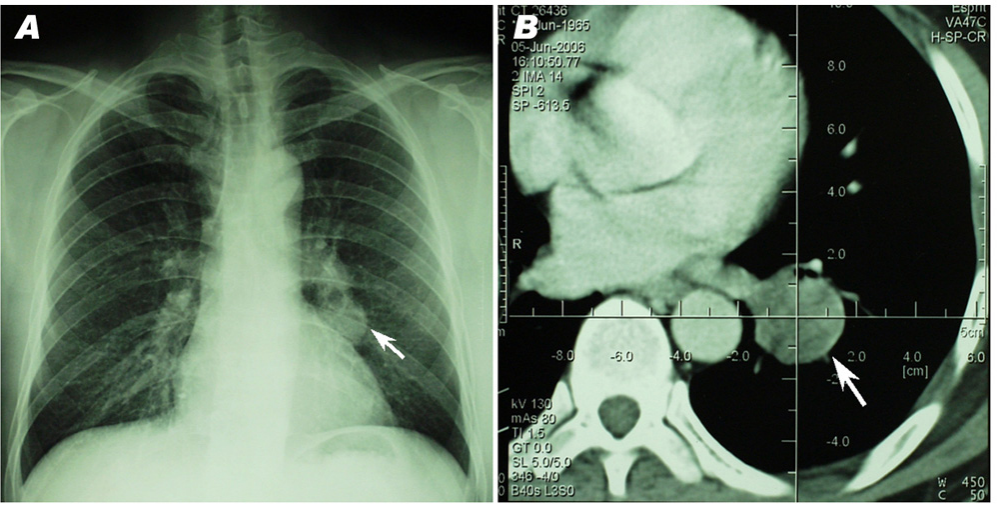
Imageological examination of one patient. Chest X-ray (A) and CT Examination (B) showed a solitary nodule over left lower lobe, with irregular margin and small lobulations, which could not be differentiated from lung cancer before operation.
